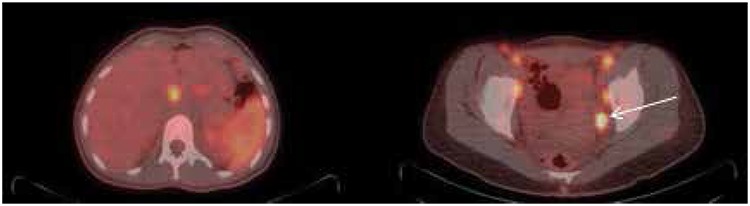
Increased fluorodeoxyglucose uptake in portocaval and external iliac chains bilaterally, most avid being the left external iliac lymph node measuring 1.7x1.5 with SUVmax: 7.5 (arrow), with associated diffuse splenic uptake
